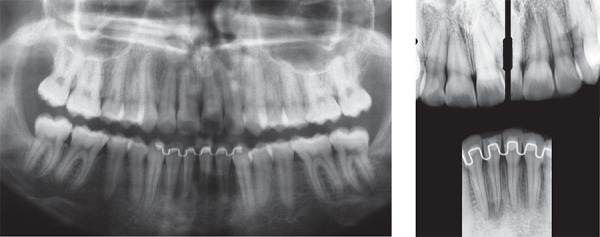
Final panoramic and periapical radiographs of incisors.
